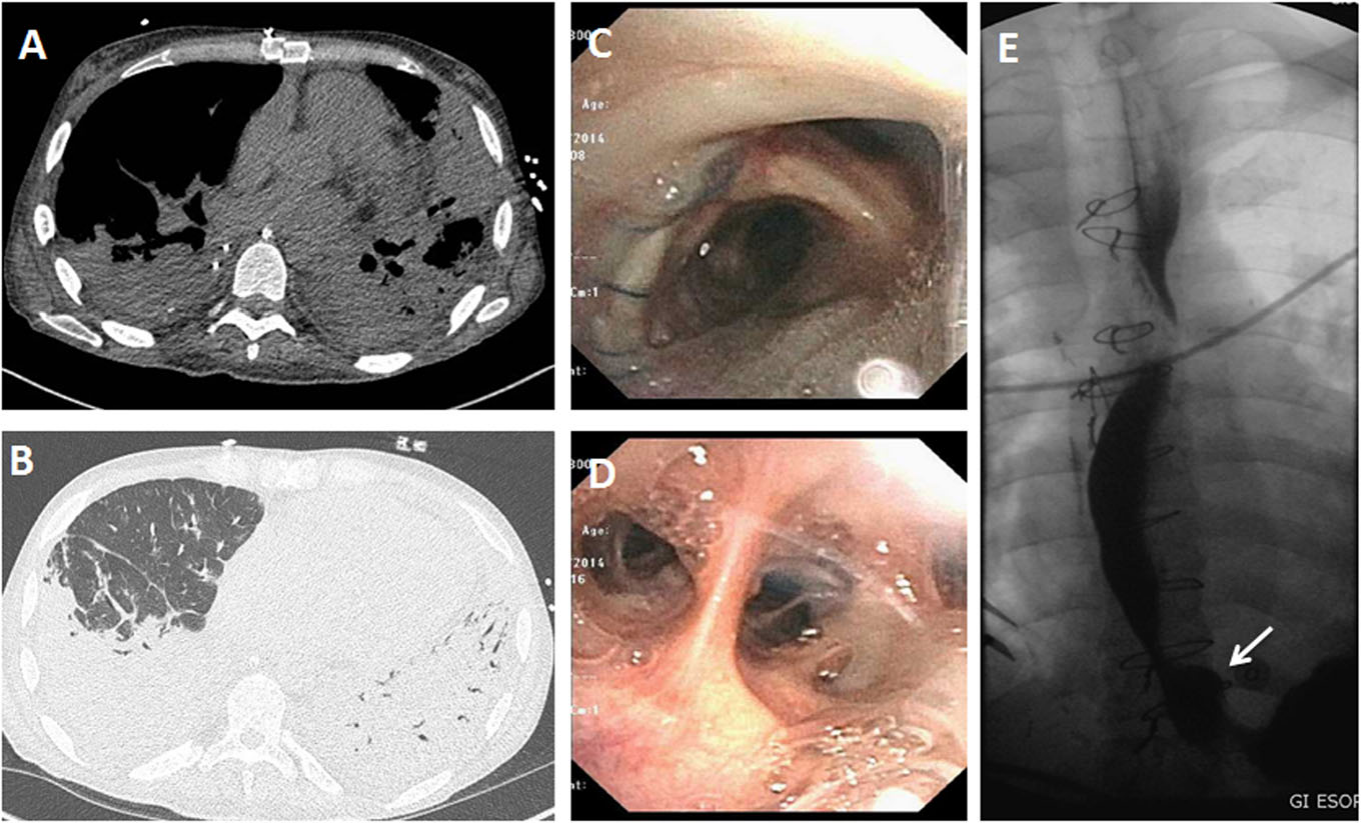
Role of multimodality imaging in a 32-year-old male status post bilateral lung transplant presenting with shortness of breath. CT images in lung window setting (a, b) show multifocal bilateral consolidations, which are predominately seen in both lower lobes. Images from flexible bronchoscopy (c, d) show mucopurulent secretions at origin of right bronchus and in right middle lobe bronchi. Still image from esophagogram (e) shows small hiatal hernia (arrow); the patient had delayed esophageal emptying. Mycoplasma IgM titers were high and the patient was felt to have mycoplasma pneumonia with co-existing aspiration pneumonia. Trans-bronchial biopsy was negative for acute rejection
